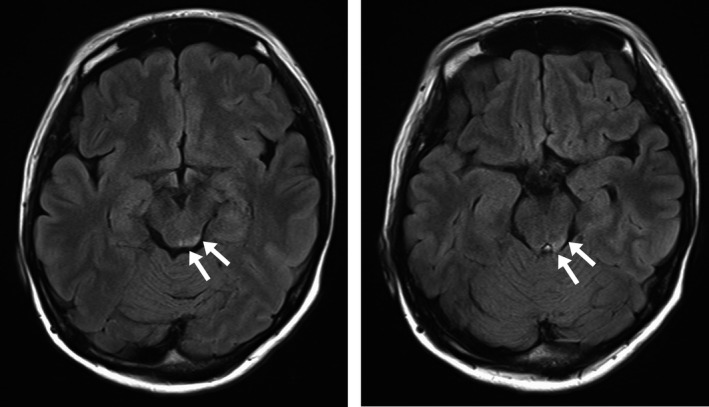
Brain magnetic resonance imaging. T2‐weighted images revealed slightly high attenuation around the inferior colliculus of the midbrain on the left side (arrows)
